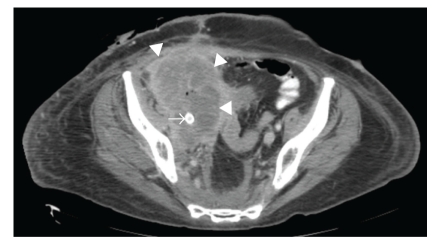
Contrast-enhanced CT scan, obtained four months after-stent insertion, demonstrates interval local tumor progression (arrowheads) but maintained patency of stented iliac artery (arrow).
